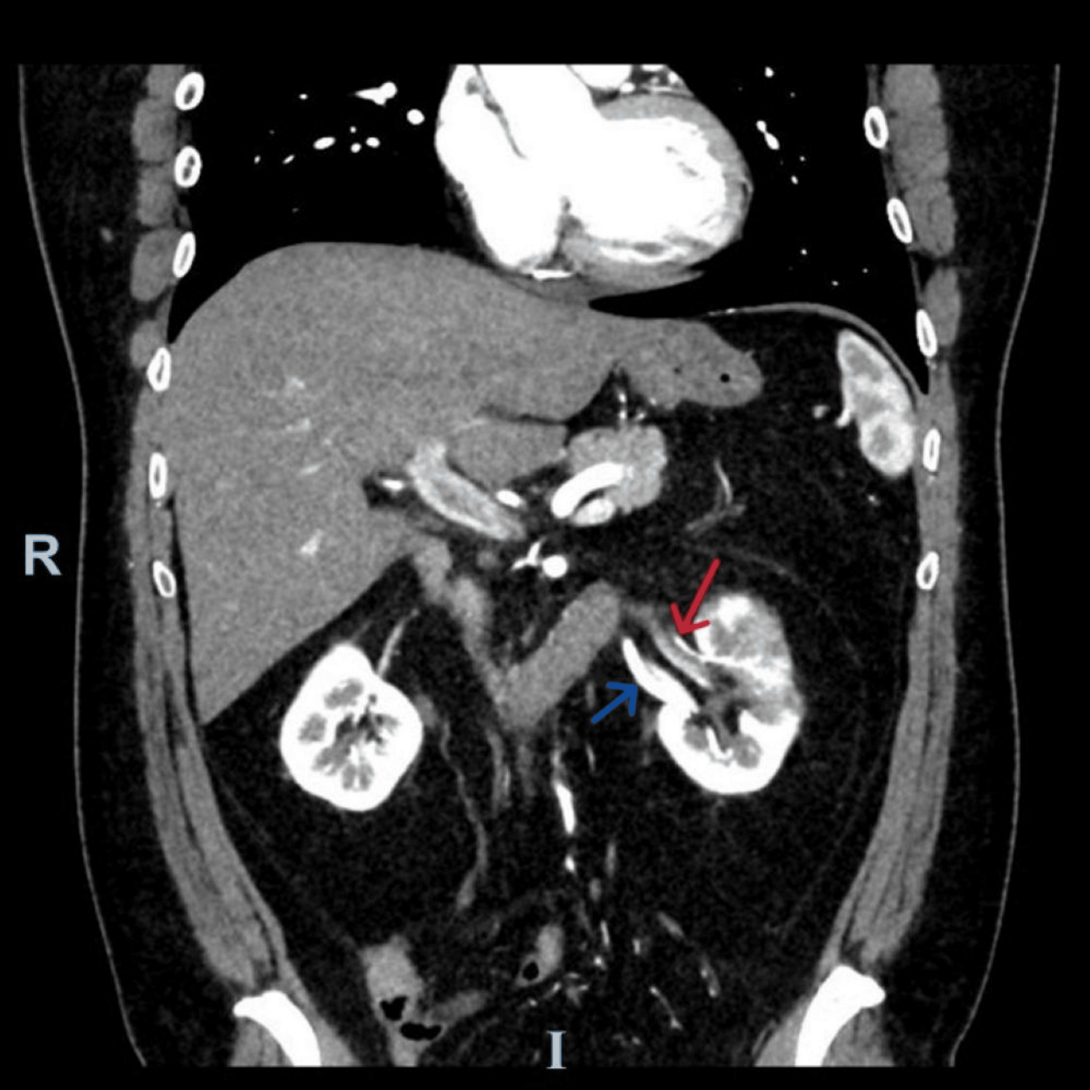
Coronal view computed tomography of the abdomen and pelvis with intravenous contrast in the arterial phase showing the two left renal arteries Red arrow: The main renal artery supplies the upper and mid poles and demonstrates multiple filling defects within its lumen. Blue arrow: The accessory renal artery supplies the lower pole and appears to be patent.
